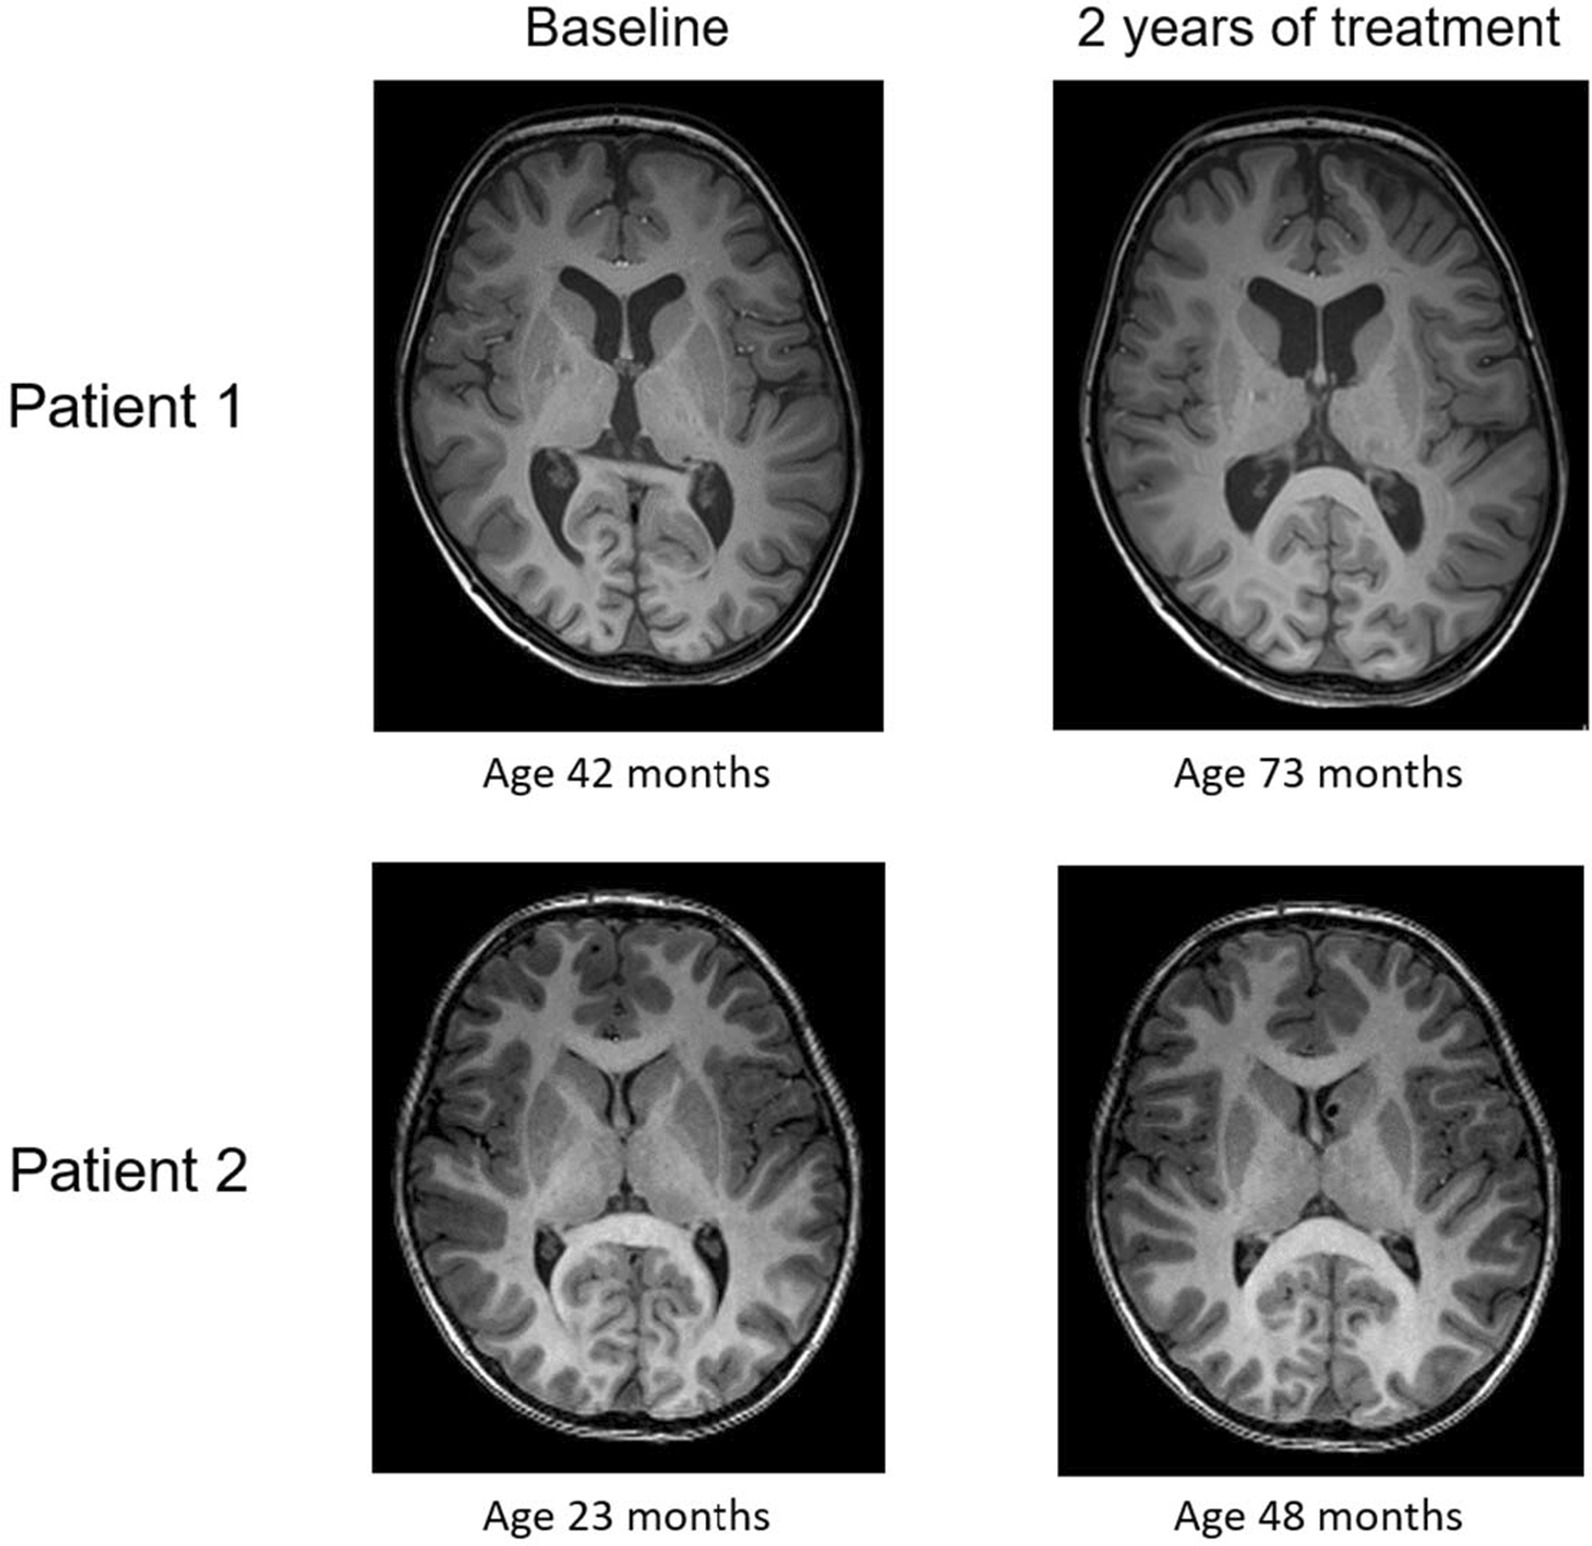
Brain MRI at baseline and after approximately two years of treatment with cerliponase alfa. Axial T1-weighted MR images at the level of the lateral ventricles. In patient 1 cerebral atrophy with ex vacuo ventricular dilatation was already present at 42 months of age (baseline), whereas no cerebral atrophy was present in patient 2 at 48 months of age after 2 years of treatment with cerliponase alfa
